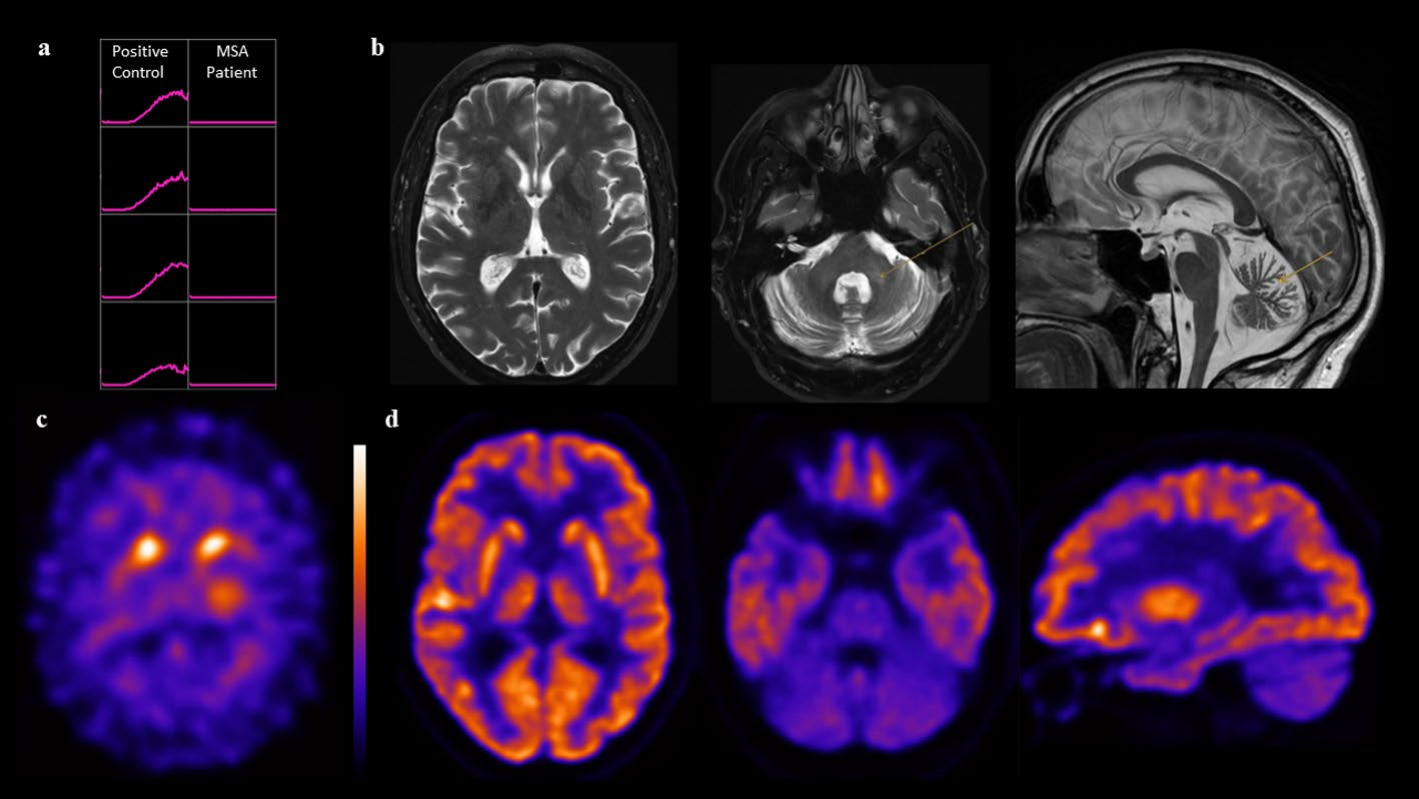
a Lewy‑fold‑specific α‑synuclein seed amplification assay (SAA) of cerebrospinal fluid (CSF) shows no detectable seeding activity in the MSA patient compared to a positive control (Parkinson’s disease). Measurements were performed in four replicates. b T2 images showing hyperintensity in the pontocerebellar tract and cerebellar atrophy (arrows, transaxial and sagittal slices) but normal putaminal signal (transaxial slice). c [^123^I]ioflupane SPECT imaging of the dopamine transporter (DAT SPECT) demonstrate severe nigrostriatal dopaminergic nerve cell loss on both sides with higher reduction in the putamen as compared to the head of the caudate (transaxial slice at striatal level) and d [^18^F]fluorodeoxyglucose (FDG) PET images (transaxial slices at striatal and cerebellar level, as well as sagittal slice at midline reveal a disease-specific uptake pattern characteristic of atypical parkinsonism primarily resembling MSA-C, but lacking the more specific feature of loss of putaminal FDG uptake indicative for MSA-P. Imaging was performed at the time of initial diagnosis (MRI) and one year later during the course of the disease (SPECT, PET), while SAA was performed consecutively to support the diagnosis of MSA
